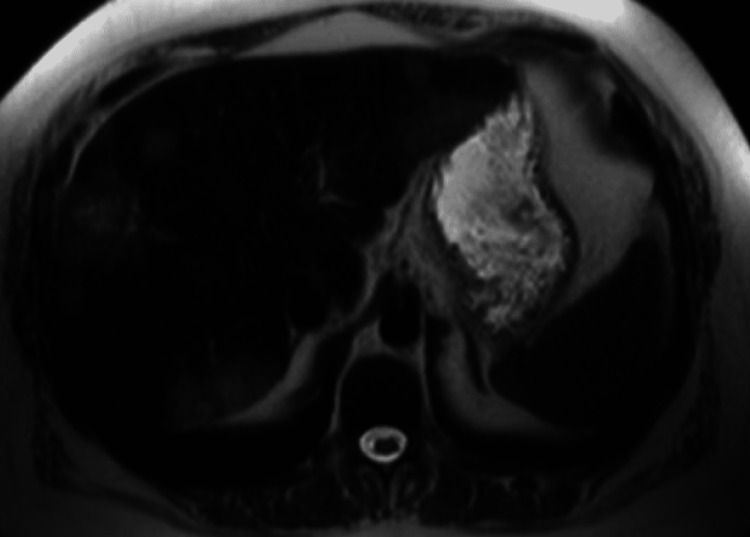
Axial view of an MRI abdomen T-2 weighted imaging showing numerous hyperintense hepatic lesions MRI: Magnetic Resonance Imaging
